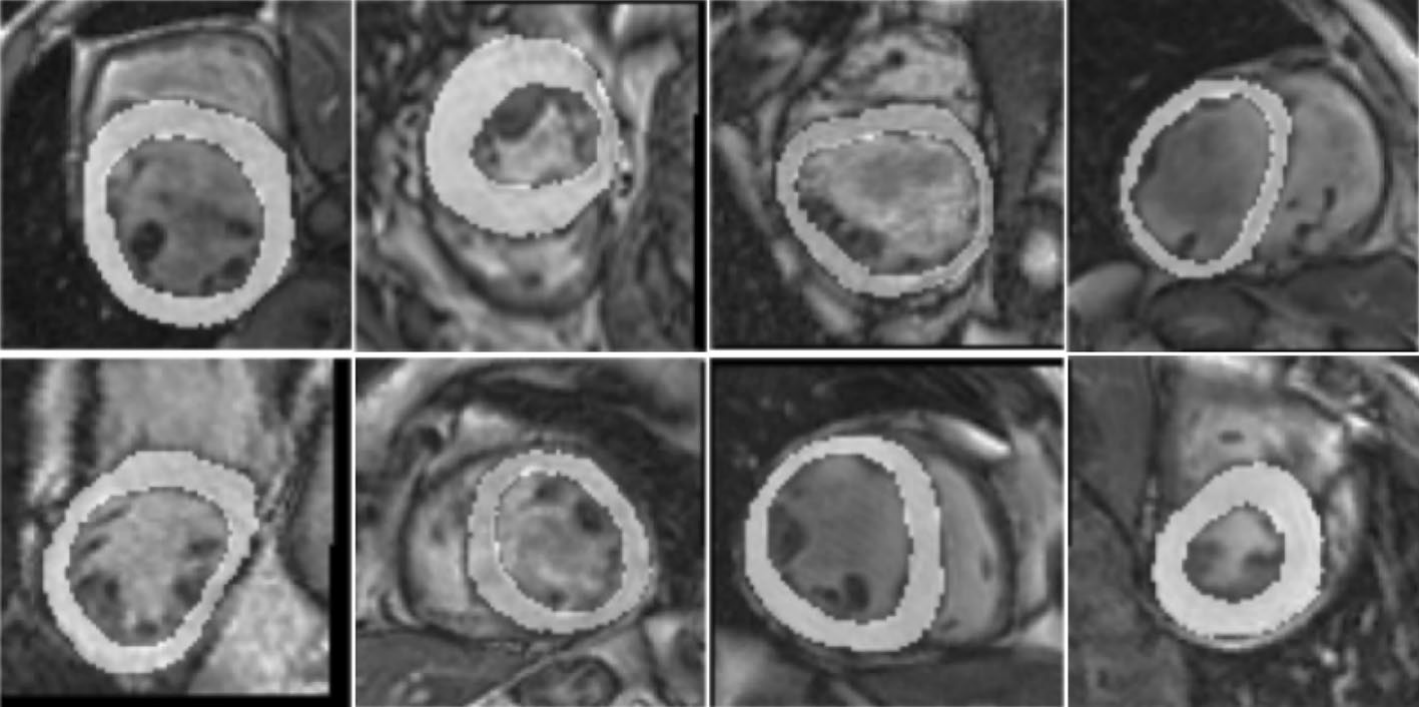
After pre-processing, we the stack the input image and its corresponding groundtruth to highlight the LV cavity and LV myocardium. From the figure, we can see the variation of shape, contrast and density in cardiac MRI. It is a great challenge for segmentation and quantification.
